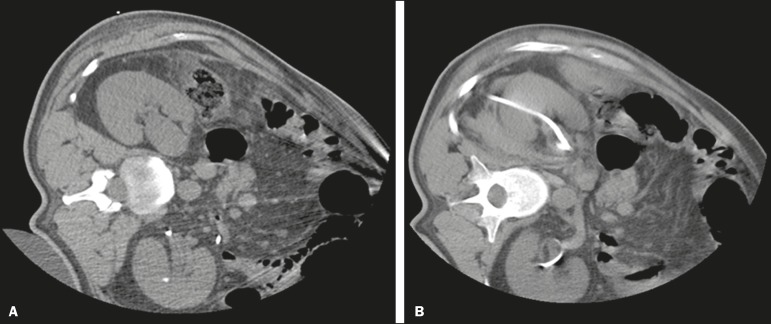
CT-guided percutaneous nephrostomy of the right kidney, by the Seldinger
